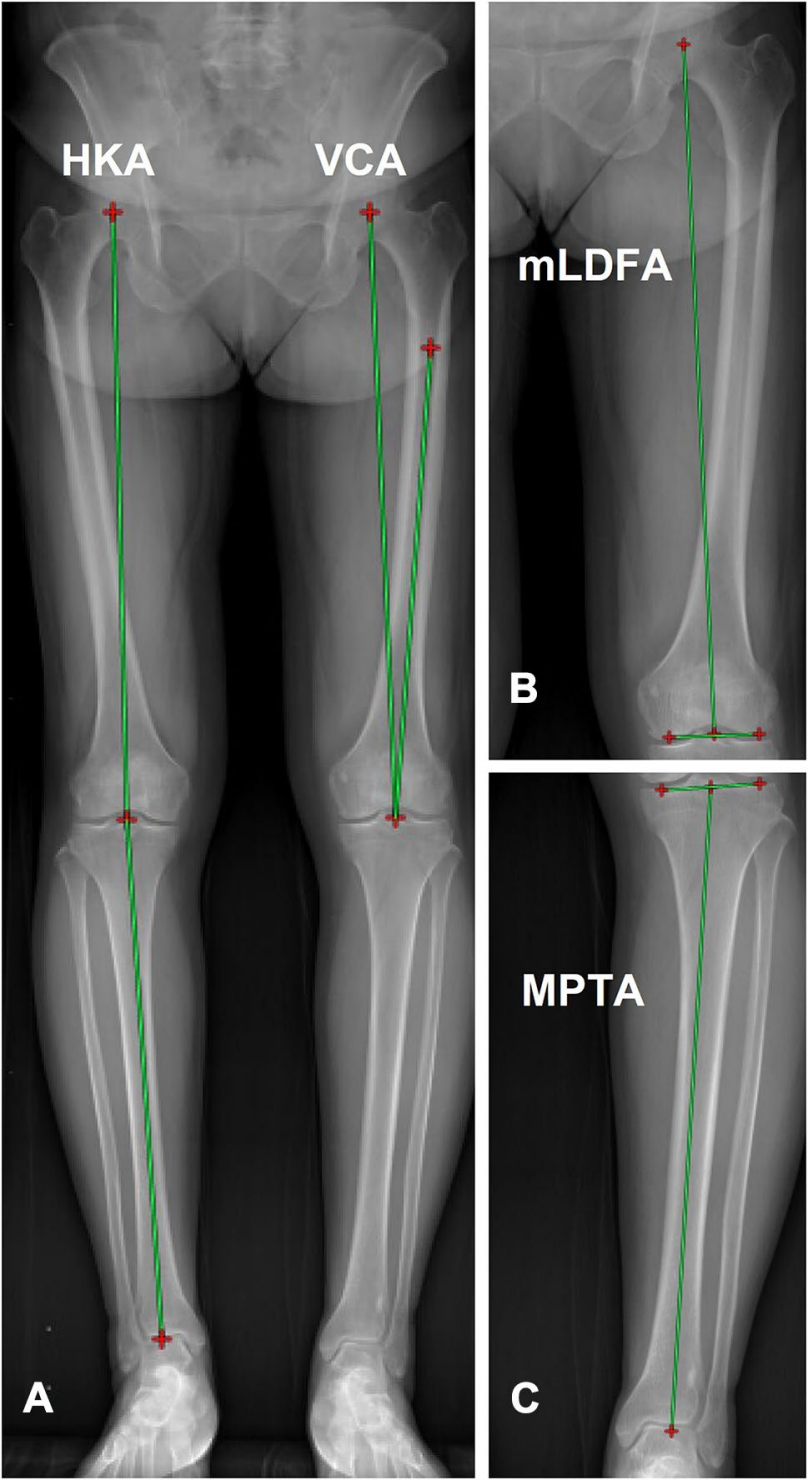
Radiographic measurements of coronal parameters. (A) Hip-knee-ankle angle (HKA) is angle between the line from the center of hip joint to the center of knee joint and the line from the center of knee joint to the center of ankle joint; Valgus correction angle (VCA) is the angle between the mechanical and anatomical axes of the femur. (B) Mechanical lateral distal femoral angle (mLDFA) is the lateral distal femoral angle between the femoral mechanical axis and the line tangential to the femoral condyles. (C) Medial proximal tibial angle (MPTA) is the medial angle between the tibial mechanical axis and the proximal tibial joint line connecting the distalmost points on the concavity of the subchondral bone of the medial and lateral tibial plateaus.
